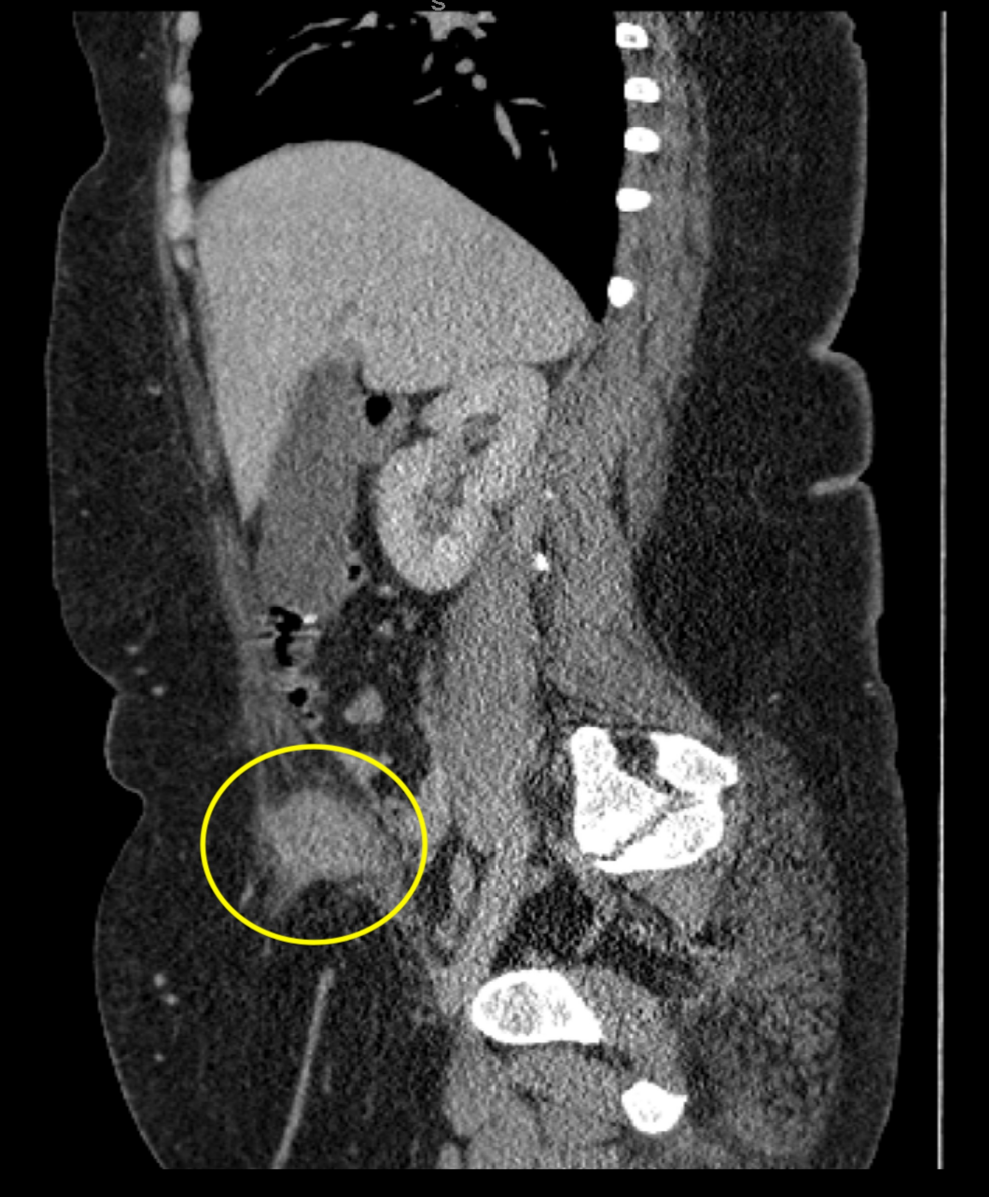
CT sagittal again exhibits an endometrioma contained in the right inferior rectus abdominus muscle, marked by an ill-defined hyperattenuating soft tissue mass (yellow circle).
